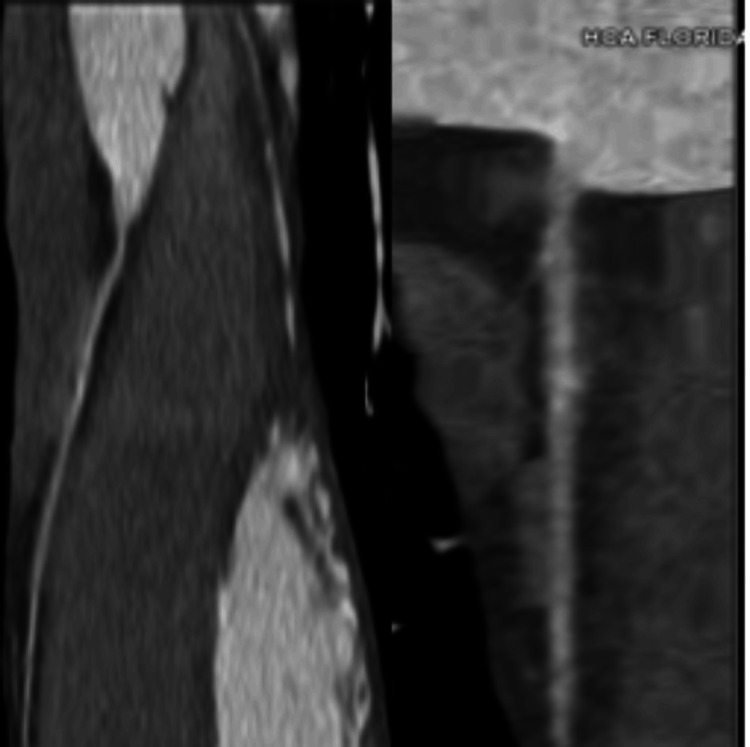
Right coronary artery. Coronary computed tomography angiography shows the right coronary artery being patent without any blockages or stenosis.
